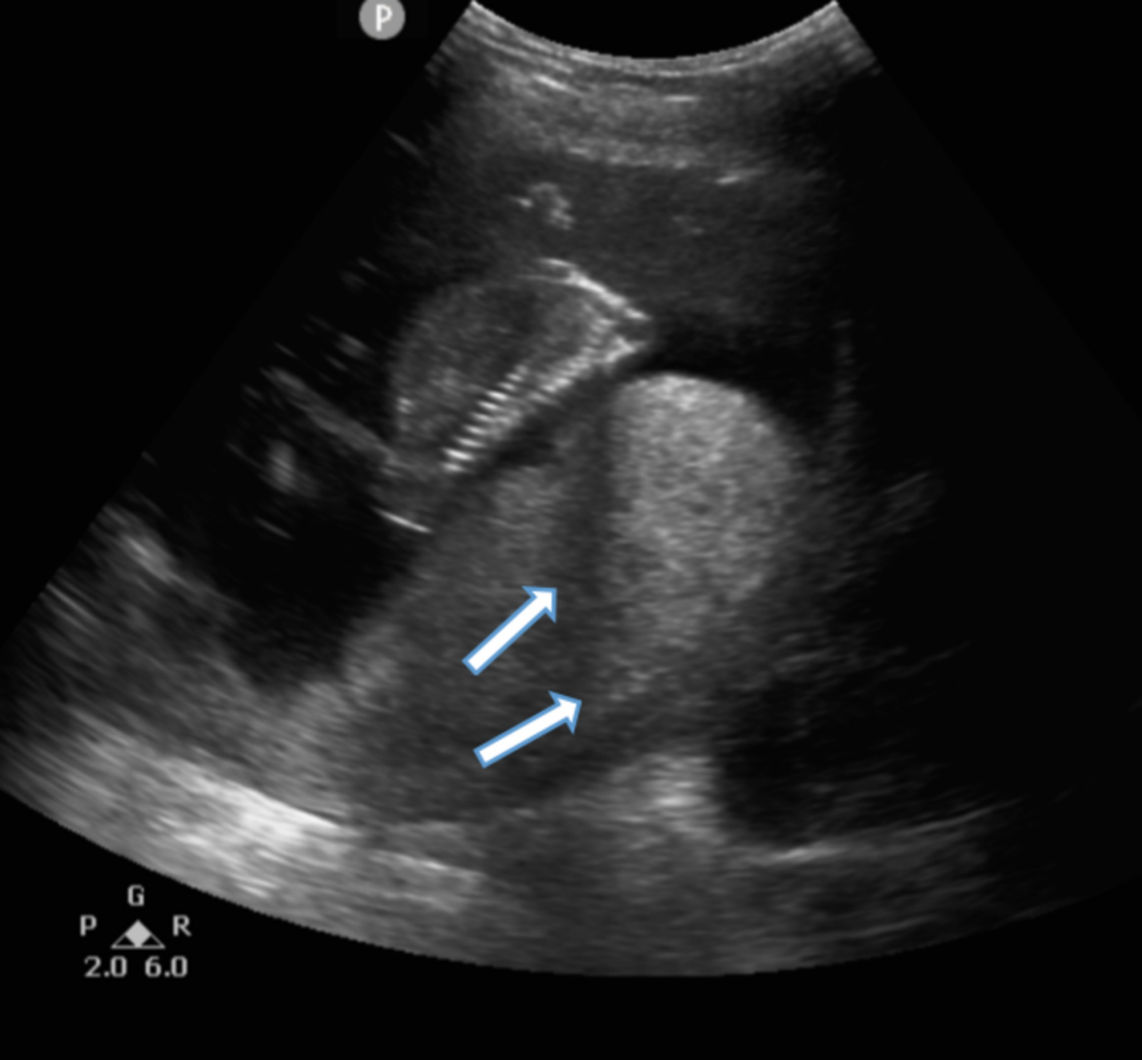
Bedside ultrasound showing intrauterine pregnancy. Bedside ultrasound of a 19-year-old gravida 2 para 1 female showing an intrauterine pregnancy with cystic tissue (arrows), posterior placenta, and present fetal heart tones (waveforms not present in the figure above).
